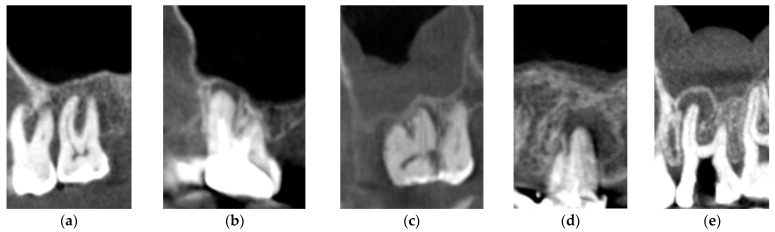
CBCT sagittal reconstructions showing the status of periapical region according to the five classes of PSI: (a). Class 1; (b) Class 2; (c) Class 3; (d) Class 4; (e) Class 5.
